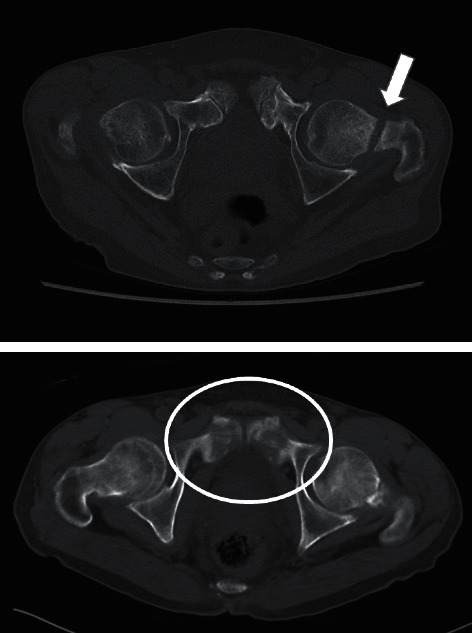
Axial view of the hip joint via computed tomography in 2016 revealing a left femoral neck fracture (white arrow). It revealed an old fracture of the pubic bone (white circle).
